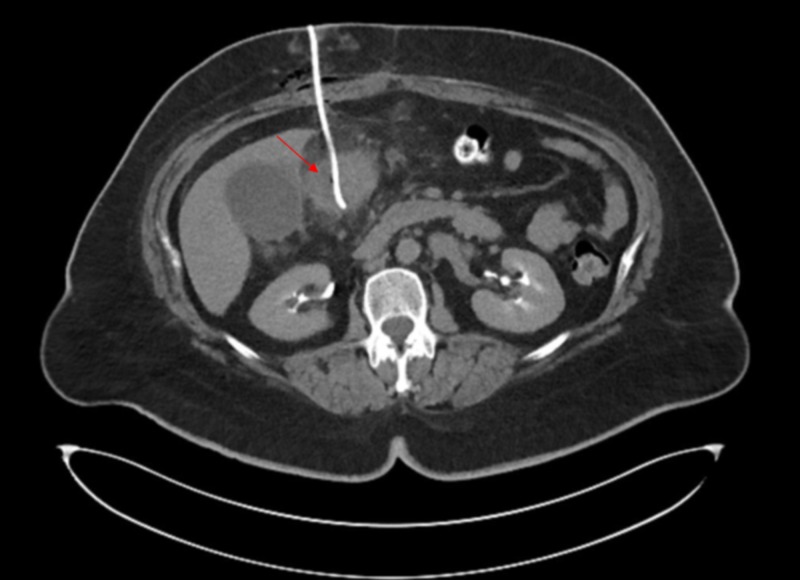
Computed tomography scan showing the pigtail catheter in place
